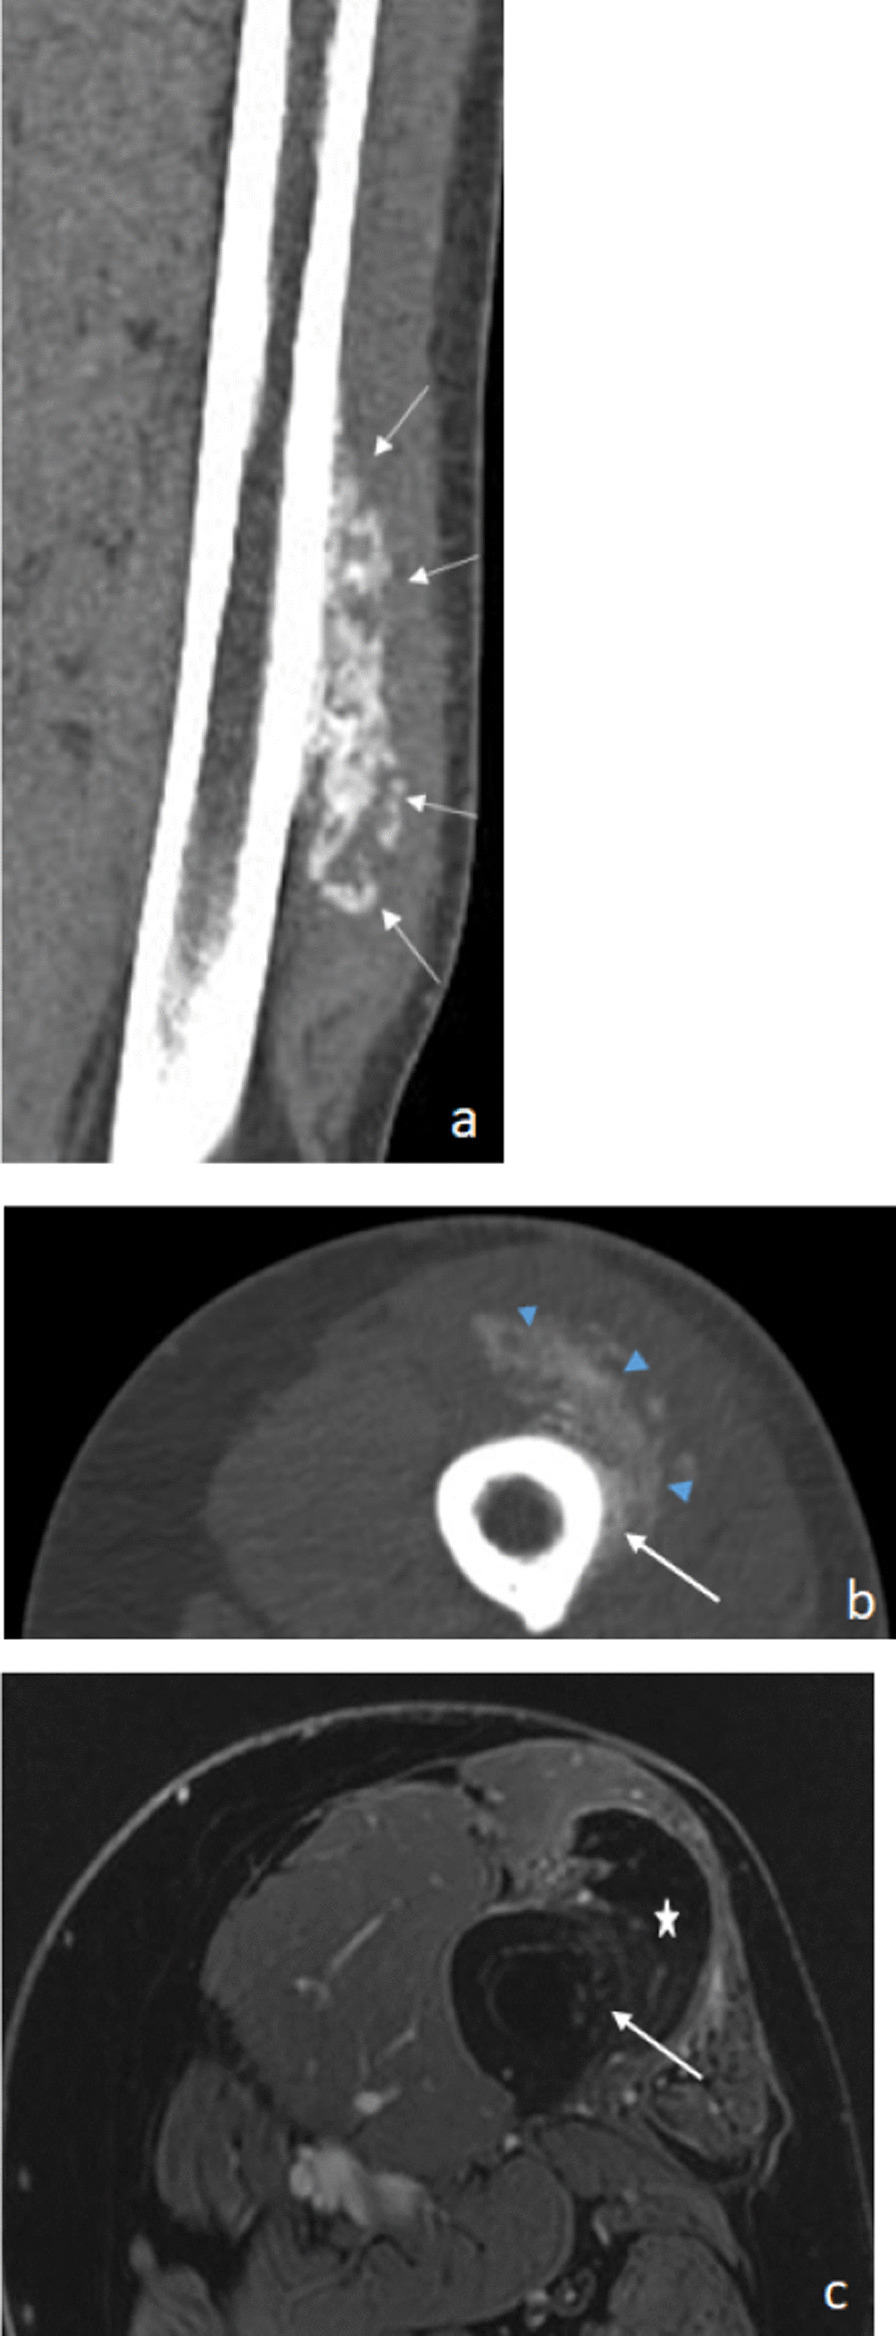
Reconstructed coronal (a) and axial (b) CT images showing an extensive calcified mass in the anterior muscle compartment (arrowhead) that is continuous with the cortical bone through periosteal ossification (arrow) in some places. Gadolinium-enhanced fat-suppressed T1-weighted MR image (c) shows a hypo-intensity T1 lesion without enhancement (star) corresponding to calcification. Note the attachment to the cortical bone (arrow). *CT* computed tomography, *MR* magnetic resonance
